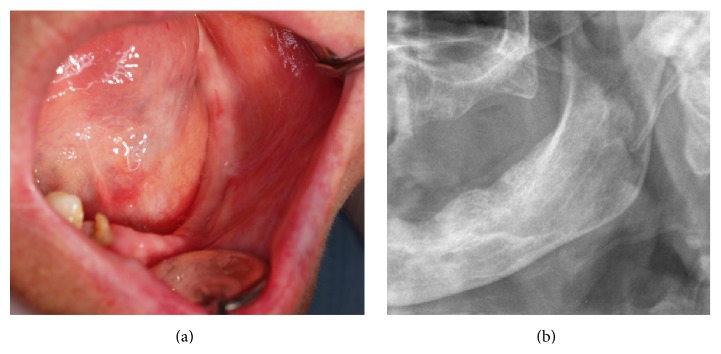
Clinical and radiographic appearance one year after excision of the lesion without signs of recurrence ((a) intraoral view and (b) panoramic radiographic image).
